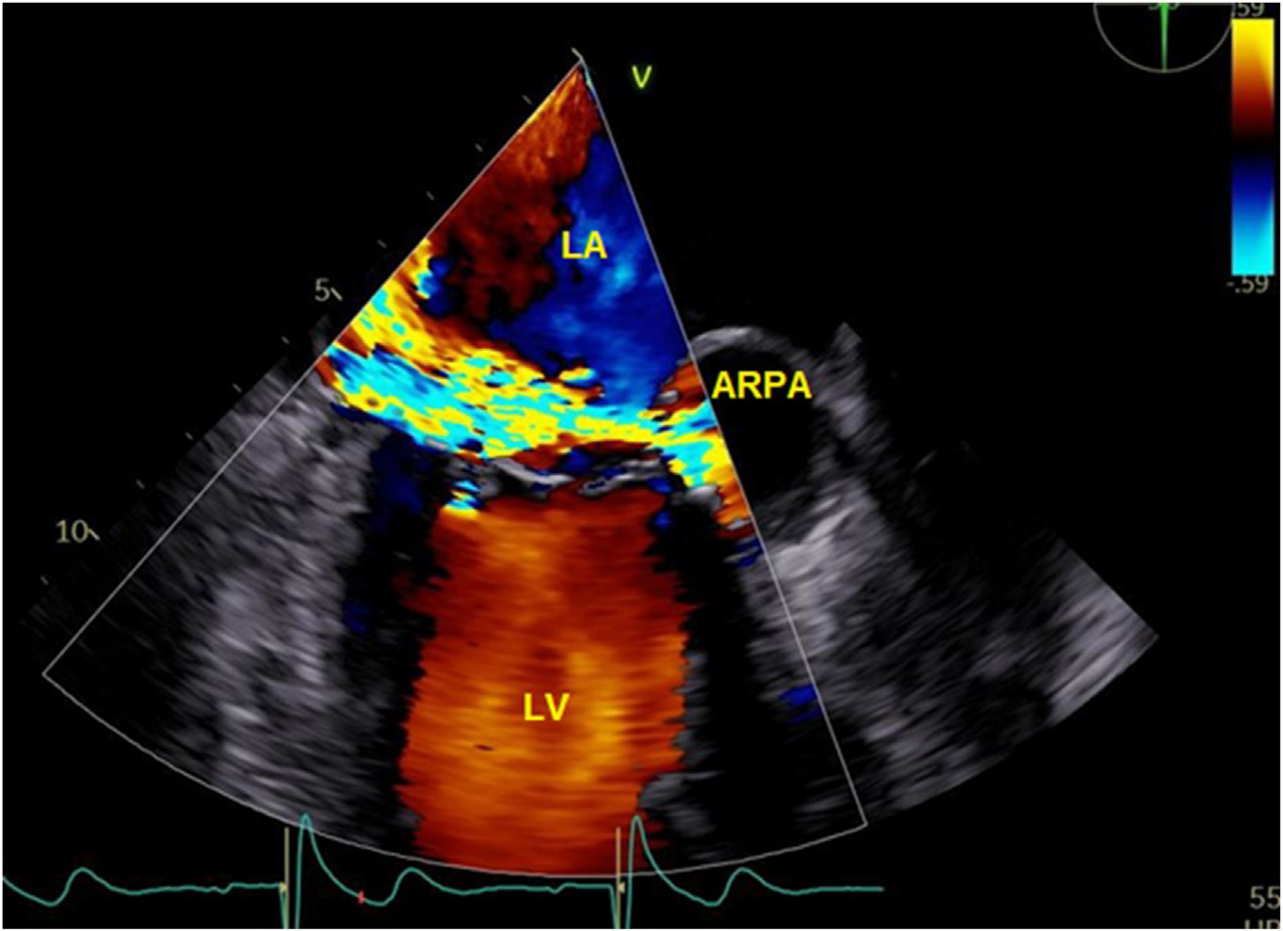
Two-dimensional TEE, mid-esophageal window, long-axis (90°) zoomed systolic display with color-flow Doppler, demonstrates the large ARPA with fistulous communication with the LA (eccentric, posteriorly directed).
